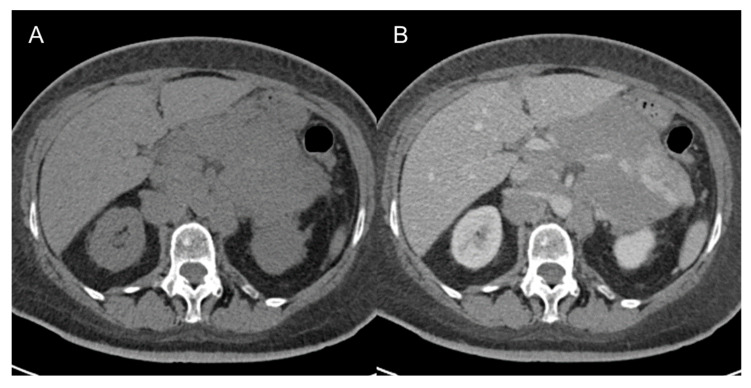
Non-Hodgkin lymphoma in 62-year-old man. Axial CT images on the pre-contrast (A) and venous (B) phases show multiple confluent retroperitoneal and mesenteric nodal masses on both sides of the upper abdominal vessels (sandwich sign).
